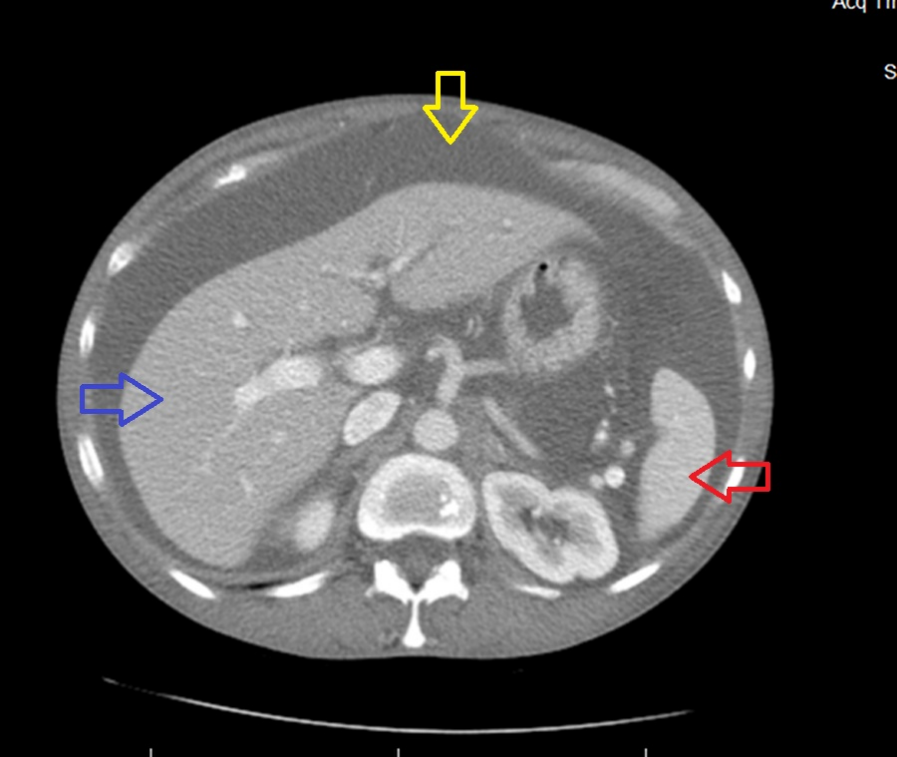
CT of the abdomen showing ascites (yellow arrow), normal sized liver (blue arrow) and spleen (red arrow)
